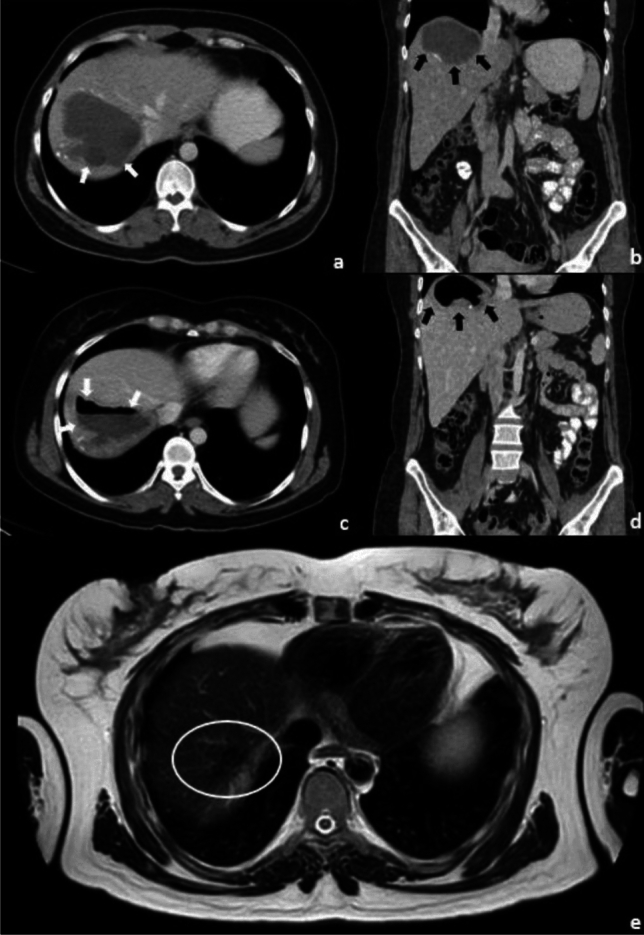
A 39-year-old female patient presented with partially peripheral calcified CE type 3b liver hydatid cyst (a and b, white/black arrows). After 9 days of the Mo-CAT procedure, in the abdominal computed tomography taken after the patient had a fever, there was an air-fluid level seen within the lesion (c and d, white/black arrows) consistent with abscesses. After effective drainage with 3 weeks and antibiotic therapy, there was no sign of active cyst or infection in T2-weighted MRI image obtained 1 year later (e, white circle) at the site of lesion but some scar tissue
